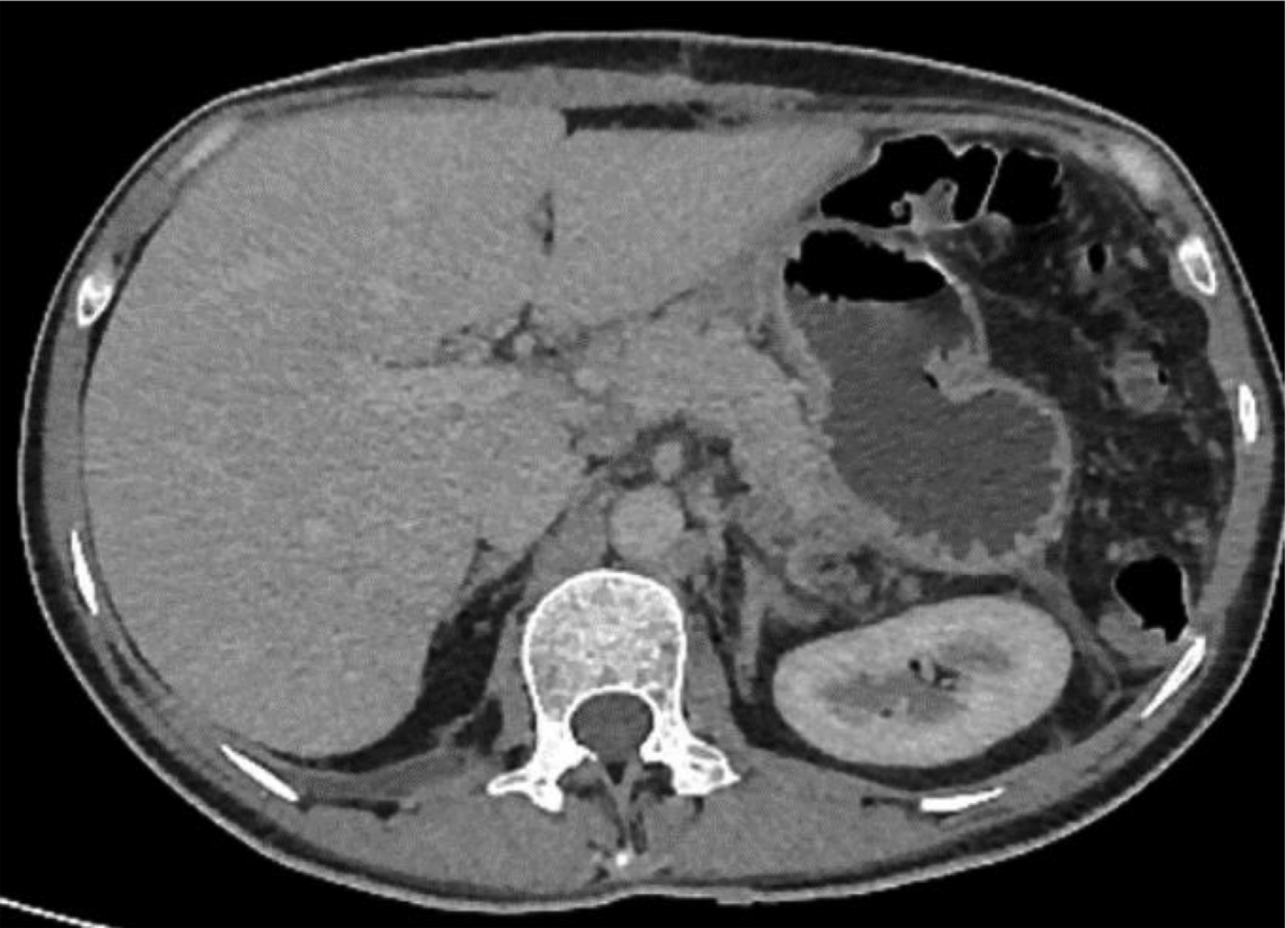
A follow-up abdomen CT scan conducted revealed resolution of perigastric and perisplenic varices.
